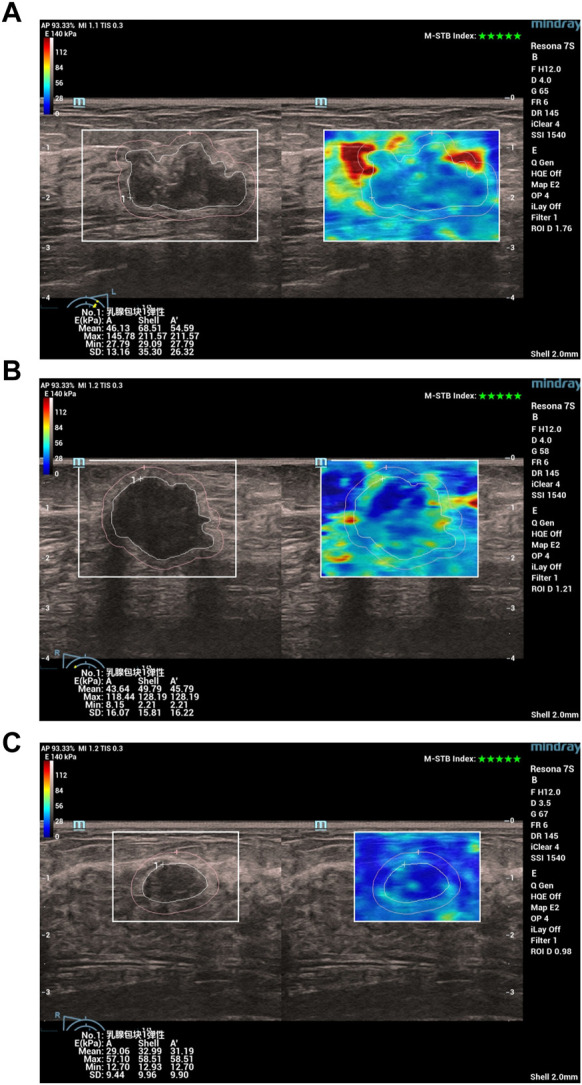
Examples of ultrasound elastography for (A) HER2+ breast cancer, (B) HER-breast cancer and (C) benign breast disease. Chinese characters 乳腺包块1弹性: in (A–C) stand for “Breast mass 1 elasticity“.
